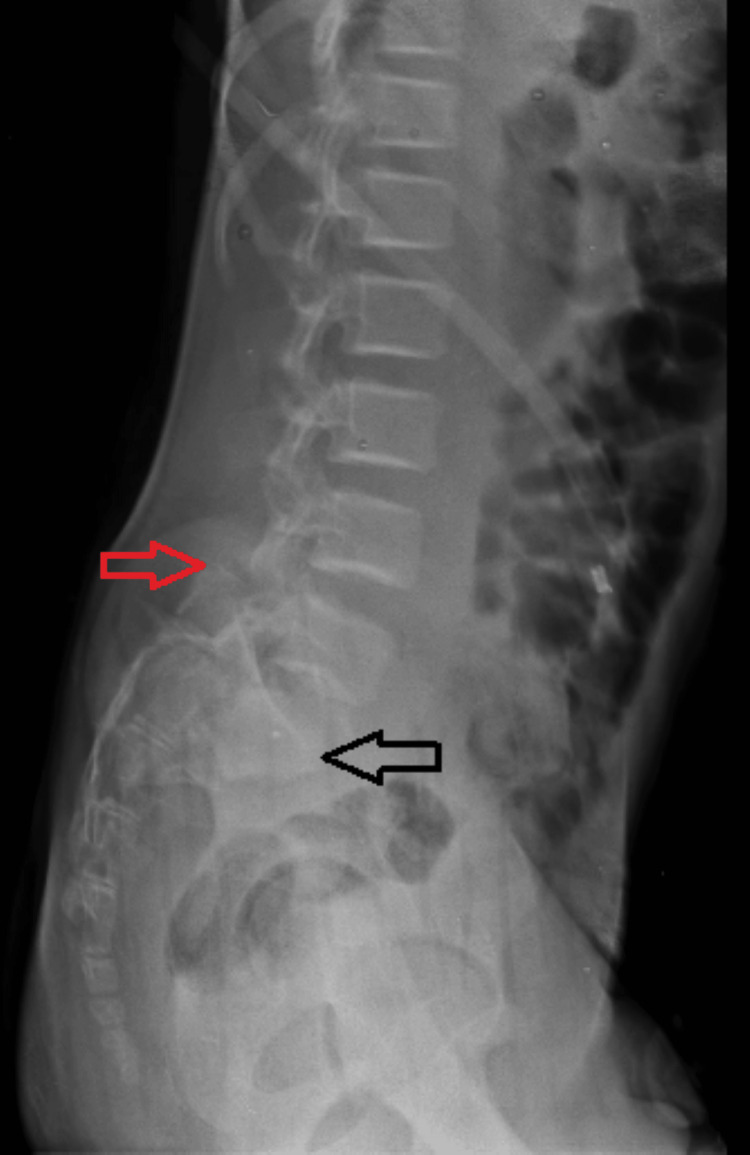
Lateral X-ray of the lumbar spine showing spondyloptosis at L5- S1 level where red arrow indicates S1 vertebra and black arrow suggests L5 vertebra
